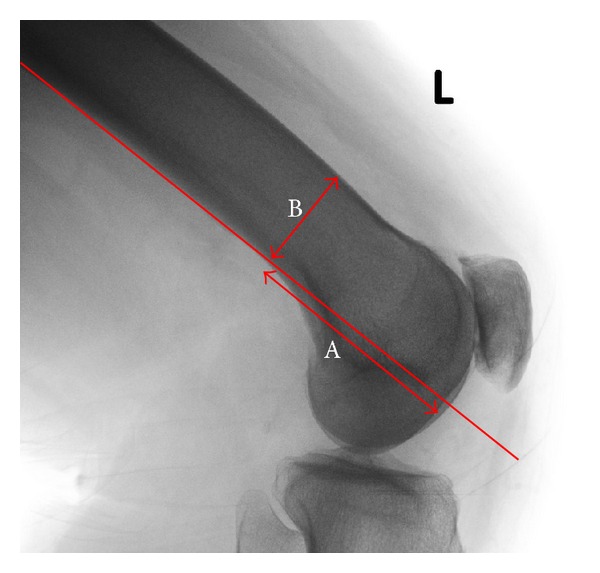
Measurement of joint line on the lateral radiograph to the distal femur. Joint line was measured (A) from the point at the tangent of the posterior cortex of the femur that crossed the flare of posterior condyles to the distal femur along that line. The joint line ratio was calculated by dividing the width of the femoral diaphysis at the level of the condylar flare (B) by the joint line (A/B).
